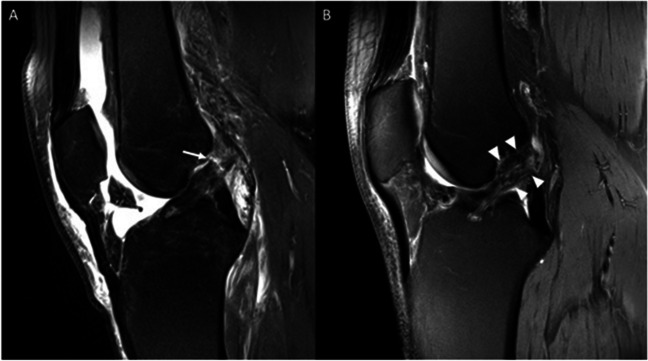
A 1.5T proton density-weighted fat-saturated MRI image of a 23-year-old male patient with a tear of the proximal ACL (arrow) after knee distortion. B 7T proton density-weighted fat-saturated MRI image of the same patient 1.5 years after primary ACL repair. The ACL appears continuous with an increased width in the proximal portion (arrowheads)
